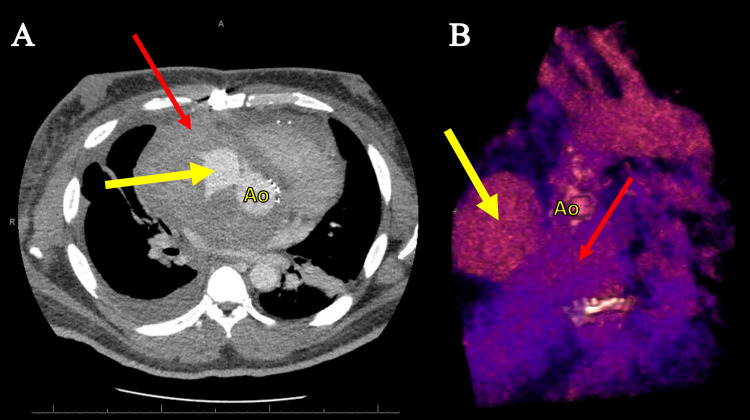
Preoperative computed tomography angiography of the chest. Large pericardial hematoma in retrosternal space (thin red arrow) with active extravasation (bold yellow arrow) from the right coronary artery re-implantation site: (A) axial view and (B) three-dimensional reconstruction.
